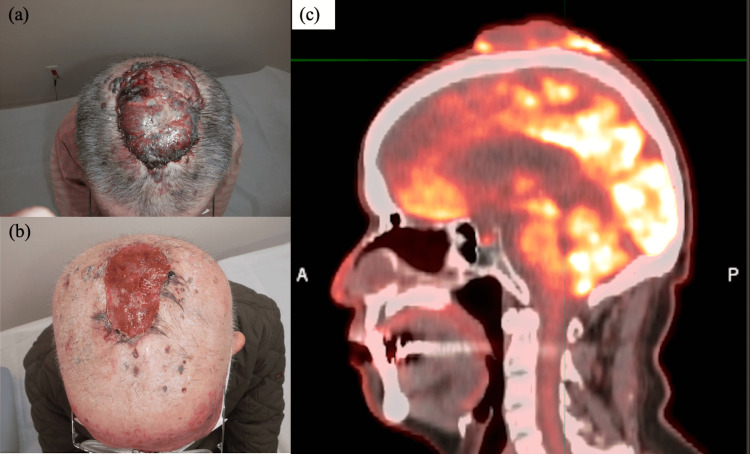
Case 1: (a) Scalp at initial examination; (b) Scalp during treatment (51Gy/17Fr); (c) Pre-treatment PET-CT. PET-CT: positron emission tomography-computed tomography
